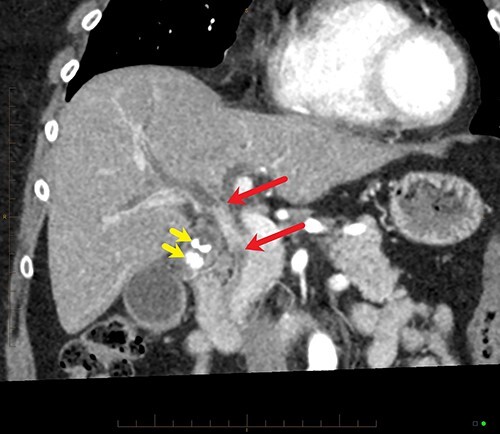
Coronal MPR of late arterial phase CT reveals segmental common hepatic duct stricture with circumferential wall thickening and enhancement (between red arrows) resulting in intrahepatic duct dilatation. Radiopaque calculi (yellow arrows) in the gallbladder.
